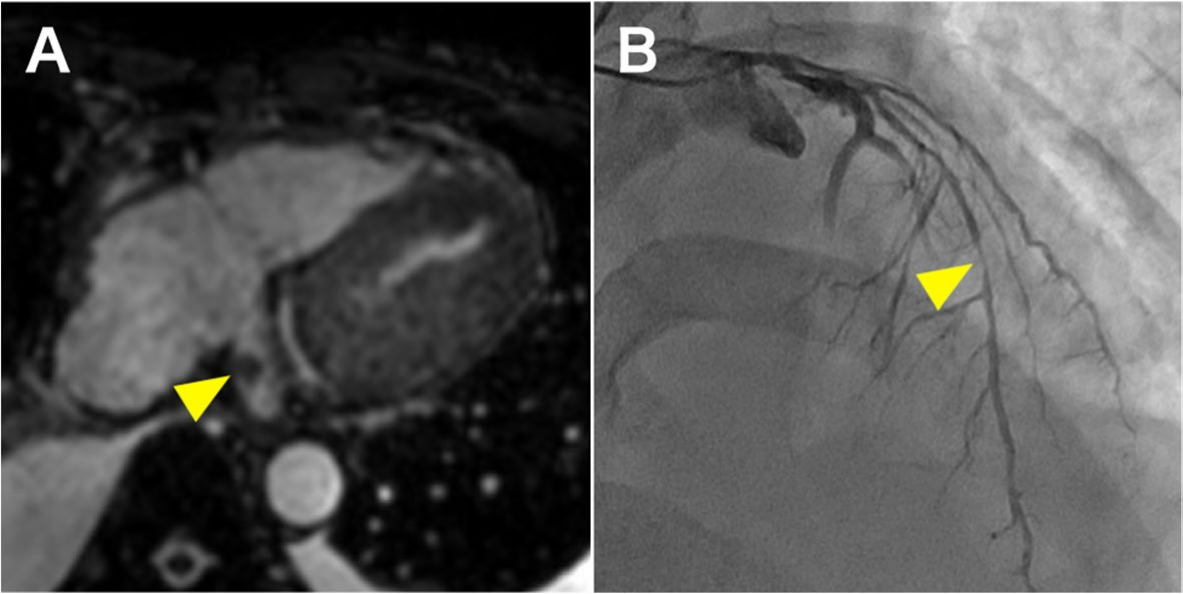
Preoperative magnetic resonance imaging and coronary angiography. A small nodule, suggesting a thrombus, was detected at the coronary sinus (A yellow arrow). There was significant stenosis in the middle of the left anterior descending artery (B yellow arrow)
